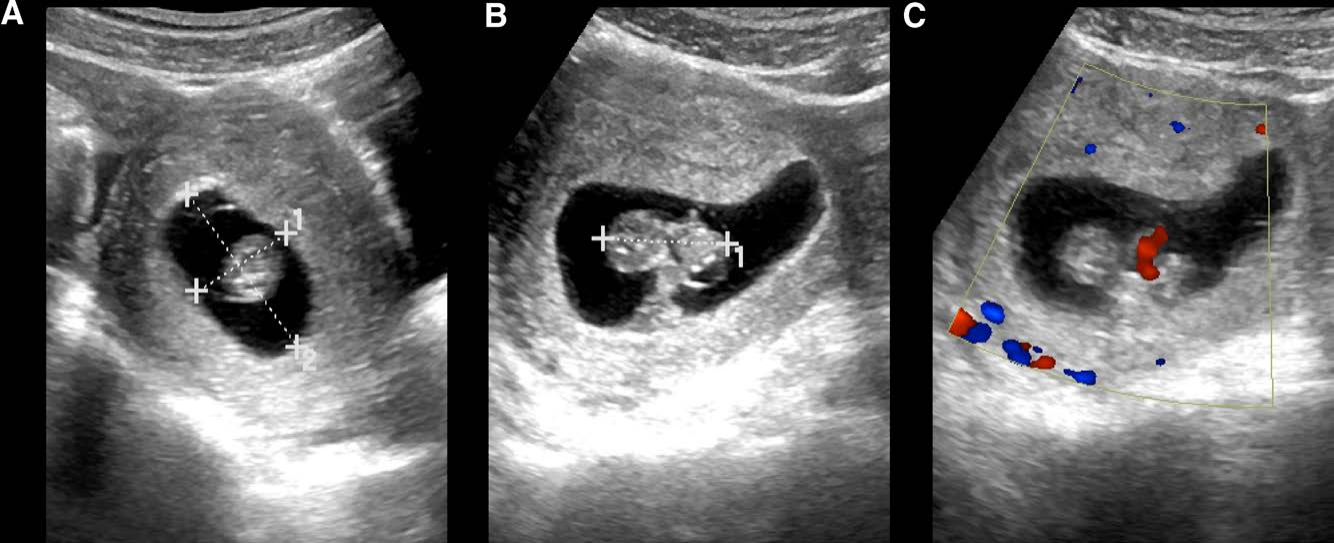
TAS results on day 66 of amenorrhea. (A) Showing IP with a gestational sac size of 55 × 24 × 42 mm. (B) Embryonic pole size of 28 mm in length. (C) CDFI revealing primitive cardiac activity within the embryonic pole. CDFI = color doppler flow imaging, TAS = transabdominal sonography.
